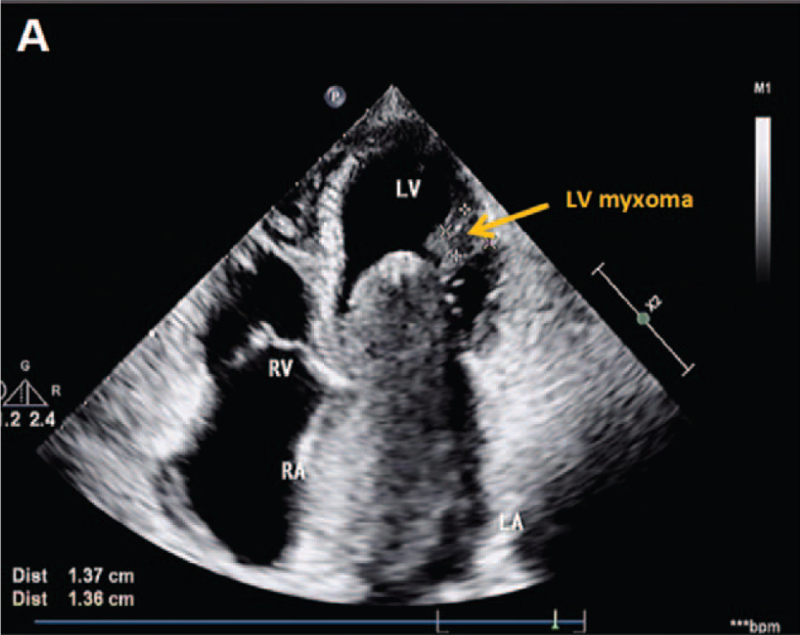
Echocardiographic imaging of LV myxoma in this patient. (A) Two-dimensional echocardiography revealed a mass of 14 mm in length and 14 mm in width (yellow arrow) in the left ventricle in the apical four chamber view. LV = Left ventricular.
